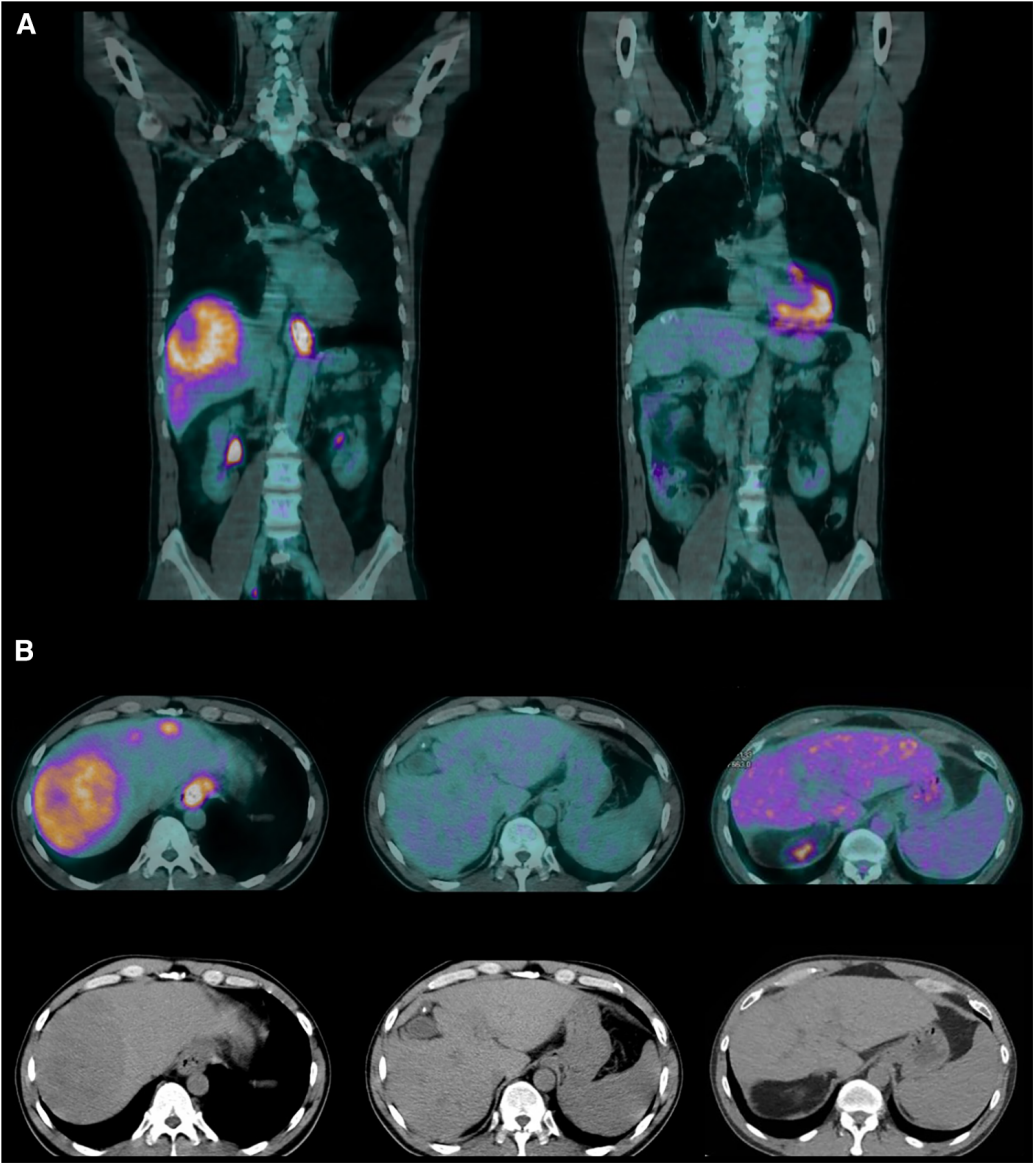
(A) Coronal PET scans (June 2019 and March 2021). (B) Axial PET and CT scans (June 2019, March 2021, and August 2022).
